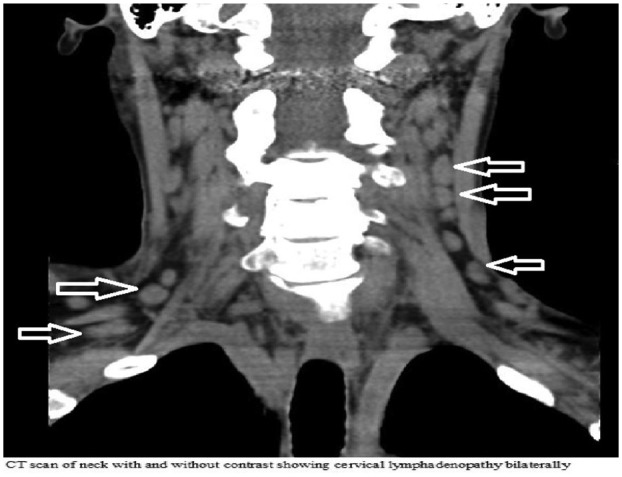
CT scan neck with and without contrast showing bilateral cervical
